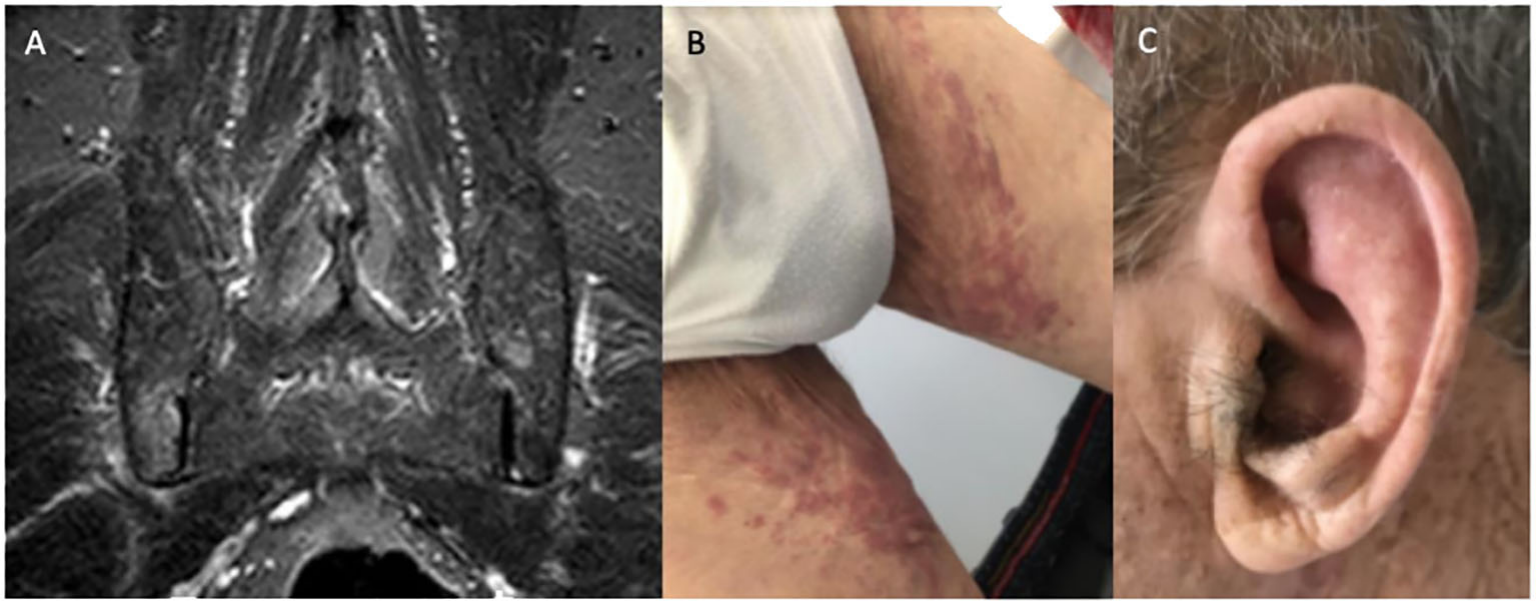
(A) Magnetic resonance imaging documenting active sacroiliitis; (B) lower limb purpuric lesions; (C) ear chondritis.
